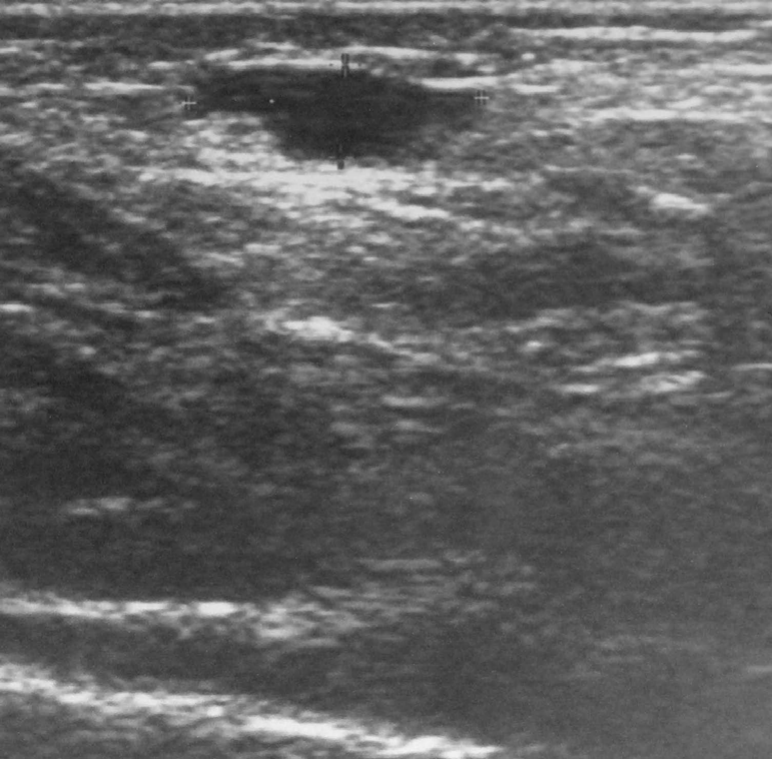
Case 1- In a six month follow-up, pre-operative Ultrasound measures the tumor 23 mm × 6 mm.
